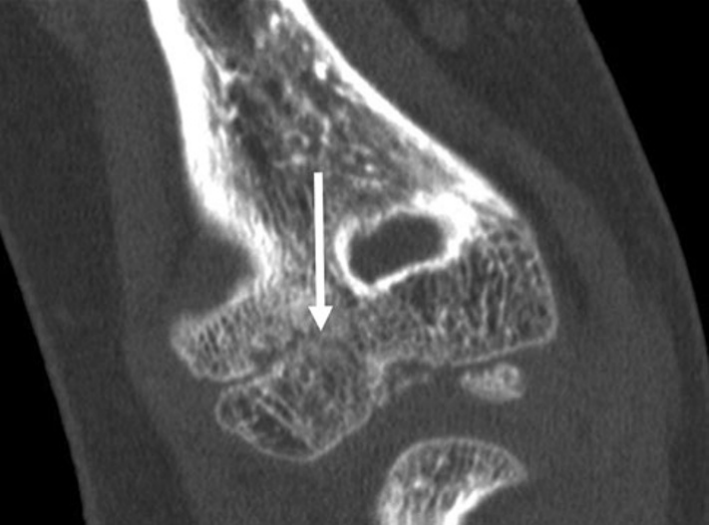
Sagital view of the CT-scan of the patients’ right elbow. The partially ossifying physis with boney bridges of the lateral humeral condyle is marked with an *arrow*
